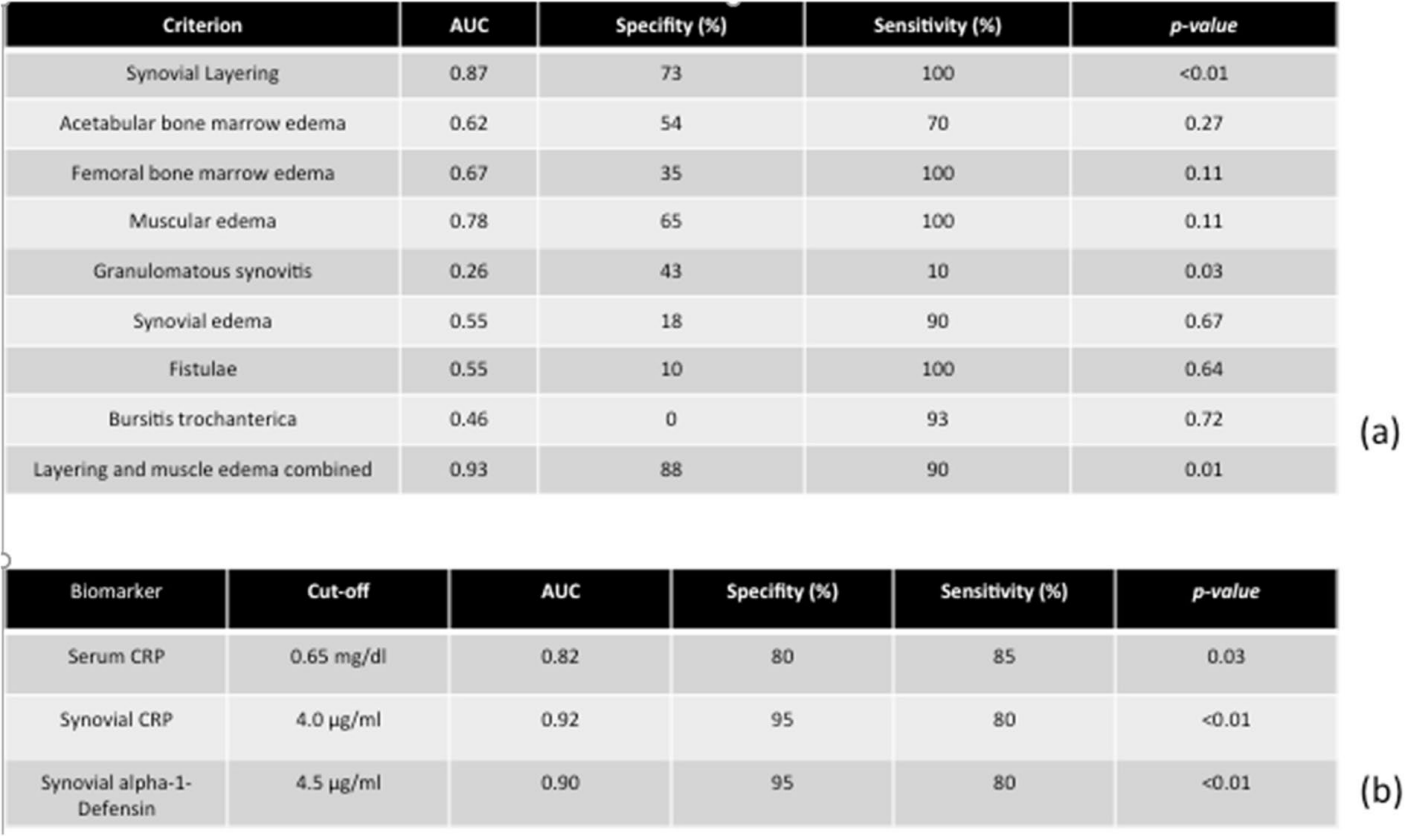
(a) Evaluation of MRI criteria for the diagnosis of periprosthetic joint infection. (b) Evaluation of serum and synovial fluid biomarkers for the diagnosis of periprosthetic joint infection
